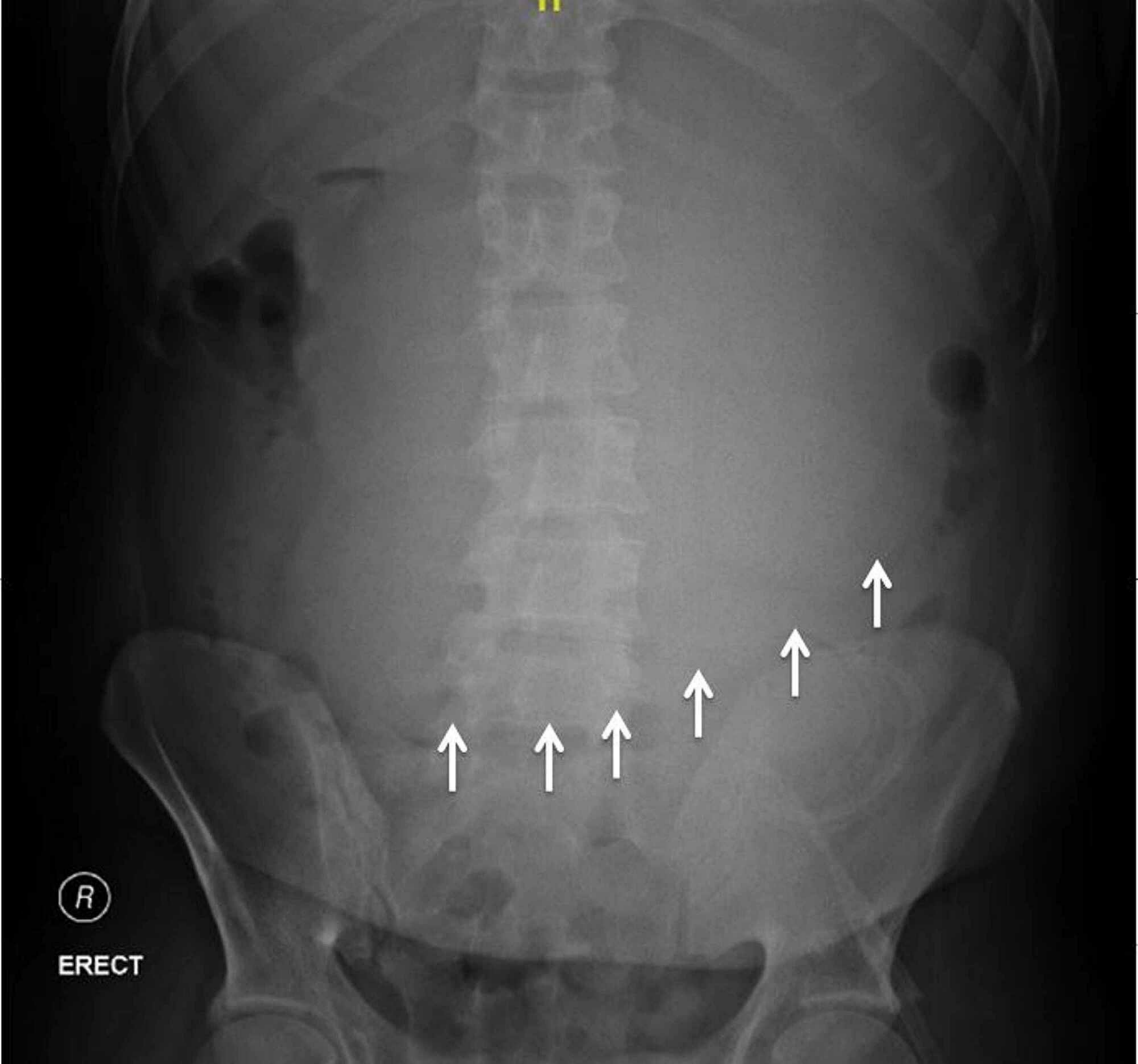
X-ray of the abdomen showing a dilated stomach (arrows).
